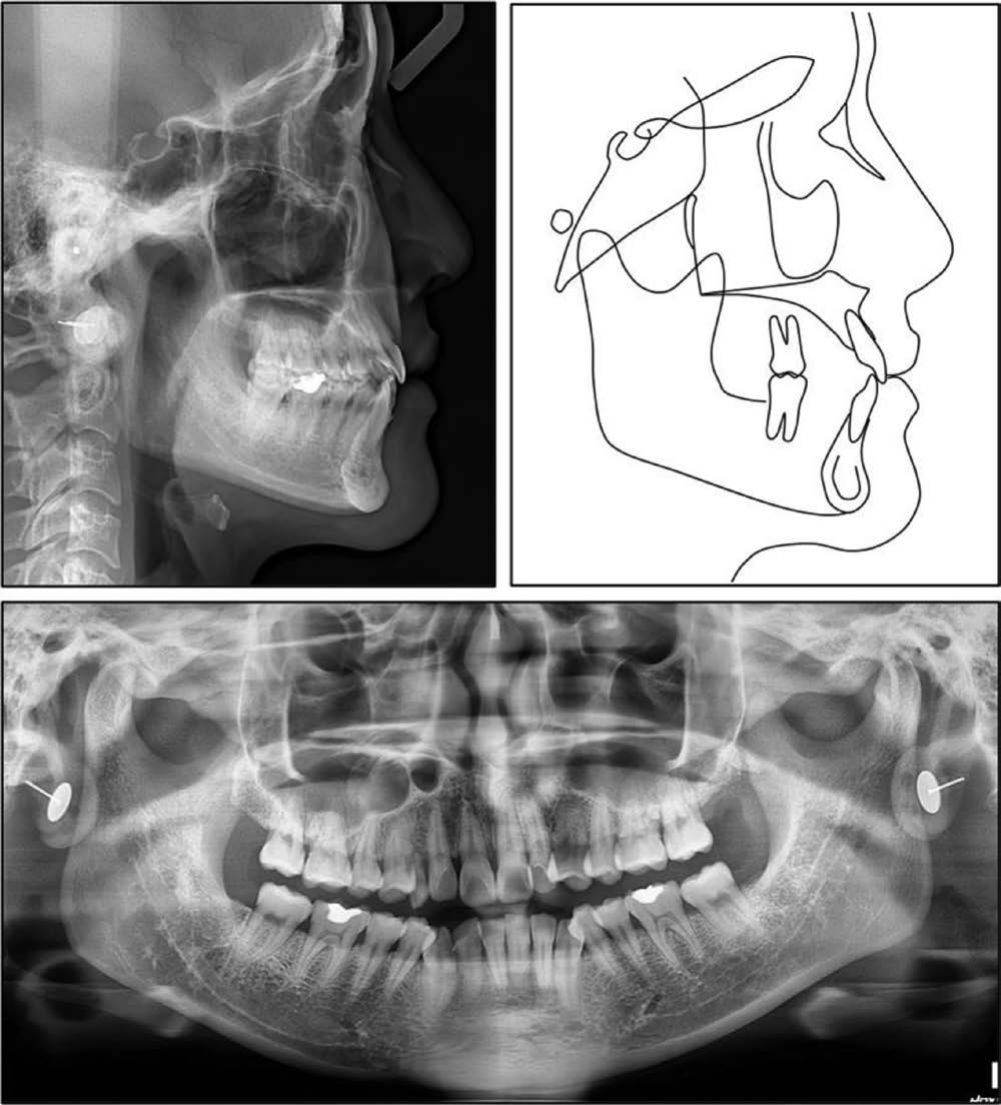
Pretreatment lateral cephalogram, cephalometric tracing, and panoramic radiograph.
